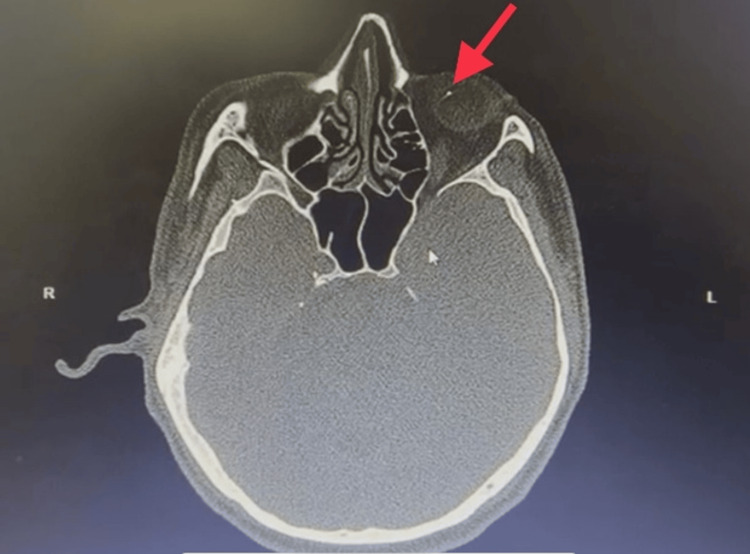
Axial view of the orbital computed tomography showing the presence of an intraocular foreign body (red arrow) located at the vitreous base.
